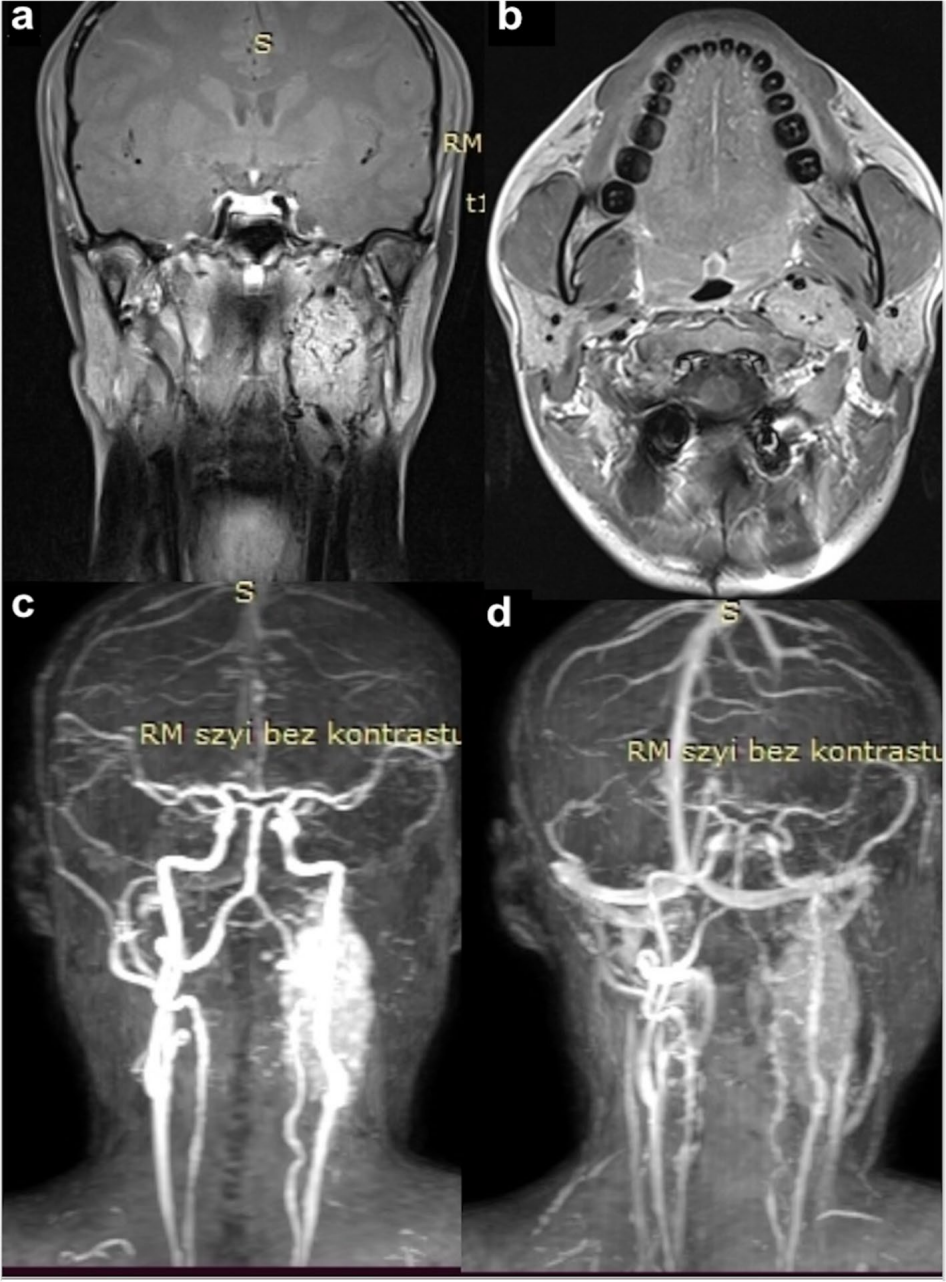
MRI and CTA imaging of a left-sided neck tumor in the patient 2. a coronal MRI, b horizontal MRI and c, d CTA scans displaying the round hypervascular tumor in the left common carotid artery bifurcation of 56 mm in the largest dimension
